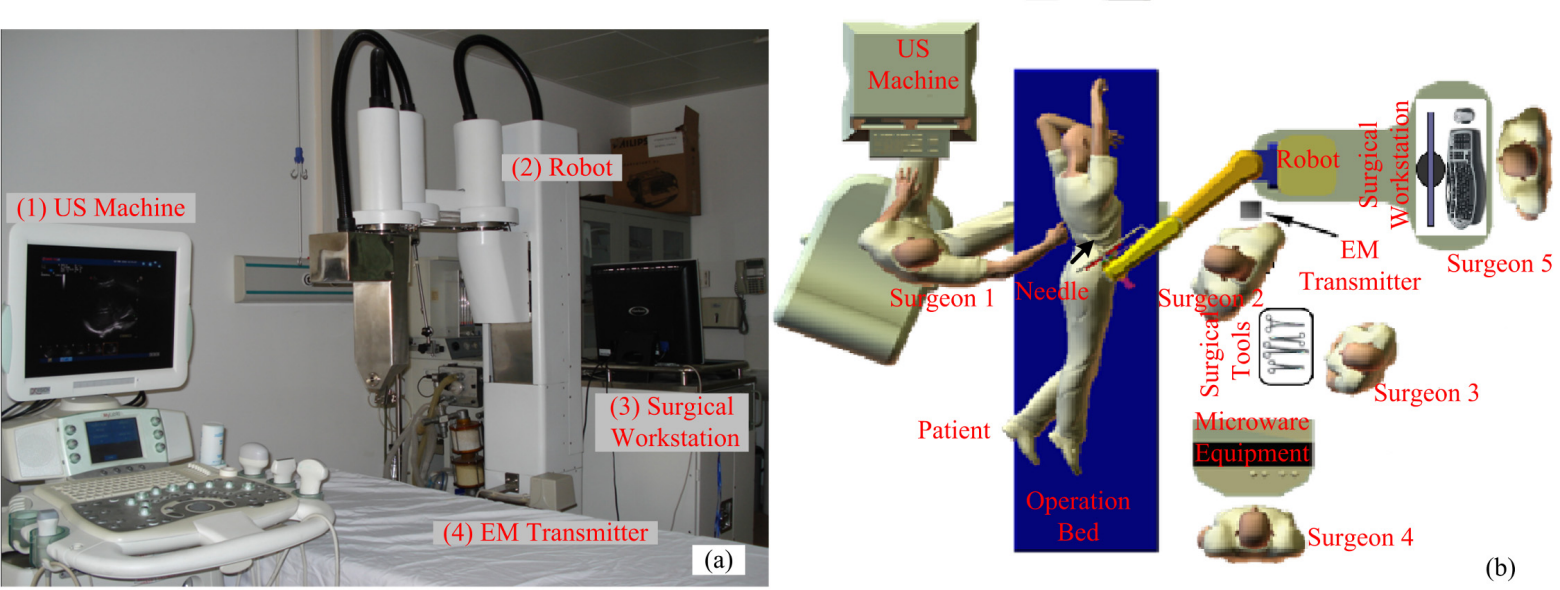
The RALTCT system and the operation environment. (a) The RALTCT system consists of four basic components: (1) the ultrasound (US) machine for two-dimensional (2D) US image display, (2) a five degrees-of-freedom (DOF) robot to manipulate the needle, (3) a PC-based surgical workstation that integrates the surgical navigation software and supervisory control of the robot, and (4) an electro-magnetic (EM) tracking system to record the position and orientation of the US probe. (b) The operation environment (top view).
